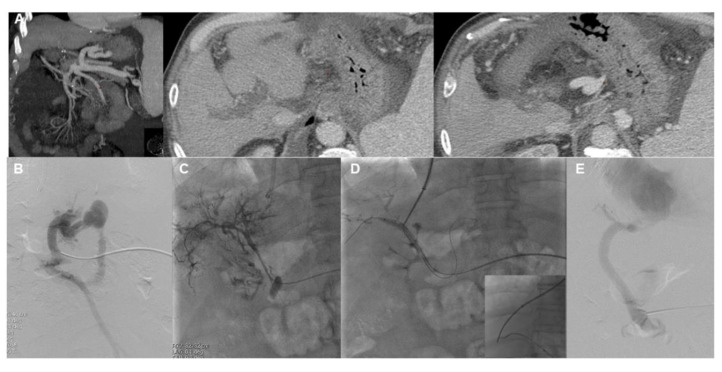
Example of a patient who developed complete intra- and extra-hepatic nonmalignant noncirrhotic portal vein thrombosis after liver transplantation. Despite receiving anticoagulant therapy, the thrombosis progressed, and the patient developed clinically significant portal hypertension, presenting with esophageal bleeding and refractory ascites. Thus, TIPS creation was pursued. (A) Abdominal CT scan showing complete intra- and extra-hepatic portal vein thrombosis and patent splenic vein. (B) The splenic vein was successfully catheterized by percutaneous US-guided puncture, and a catheter was advanced up to the spleno-mesenteric confluence. Injection of contrast shows tracking along the thrombosed portal vein. (C) An 8 mm balloon catheter was used for angioplasty and mechanical thrombolysis. (D) The ballon was then used as a landmark for portal vein puncture with a Colapinto needle, which was advanced from the right hepatic vein into a portal vein radicle, and portal system catheterization was achieved. (E) Portography performed after TIPS creation with a Viatorr 10 mm diameter e-PTFE-covered stent. Of note, there is no filling of varices at the final portography.
